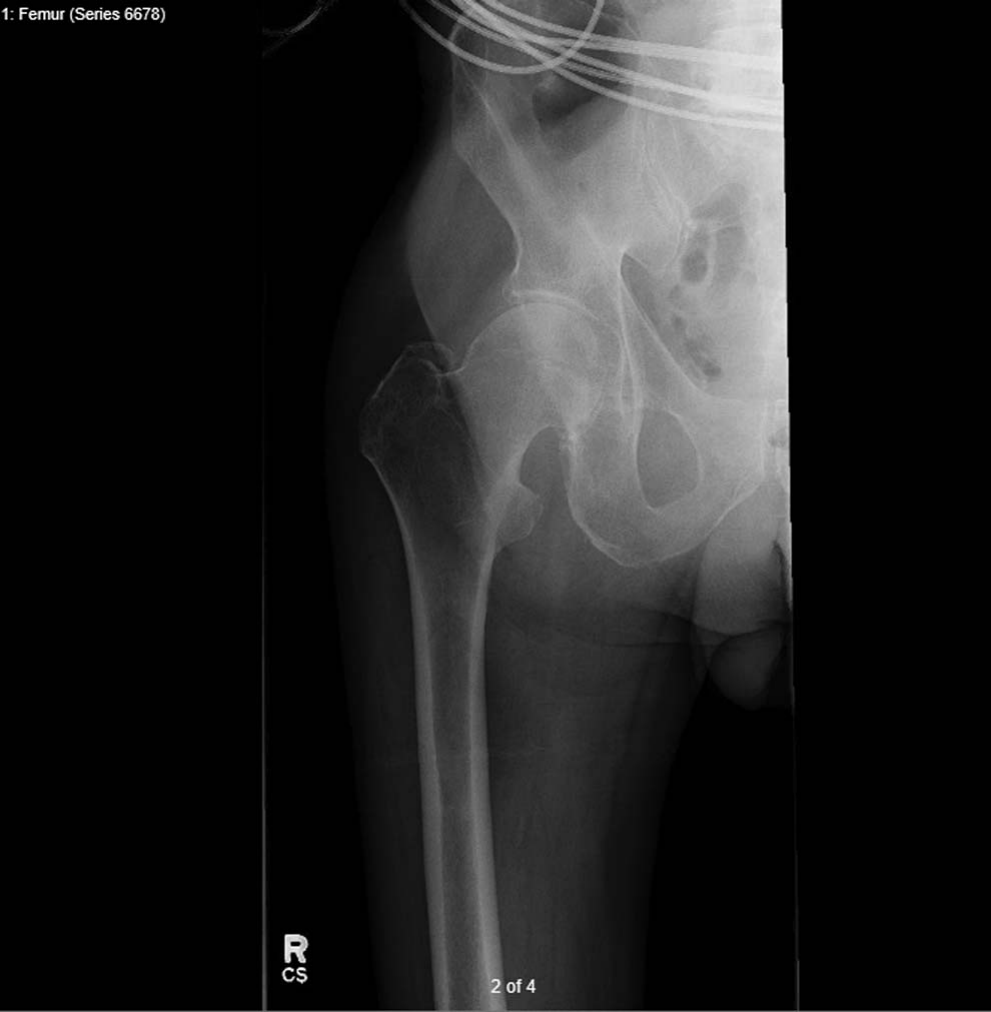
Anteroposterior x-ray of the right femur shows a possible greater trochanteric chip fracture.
